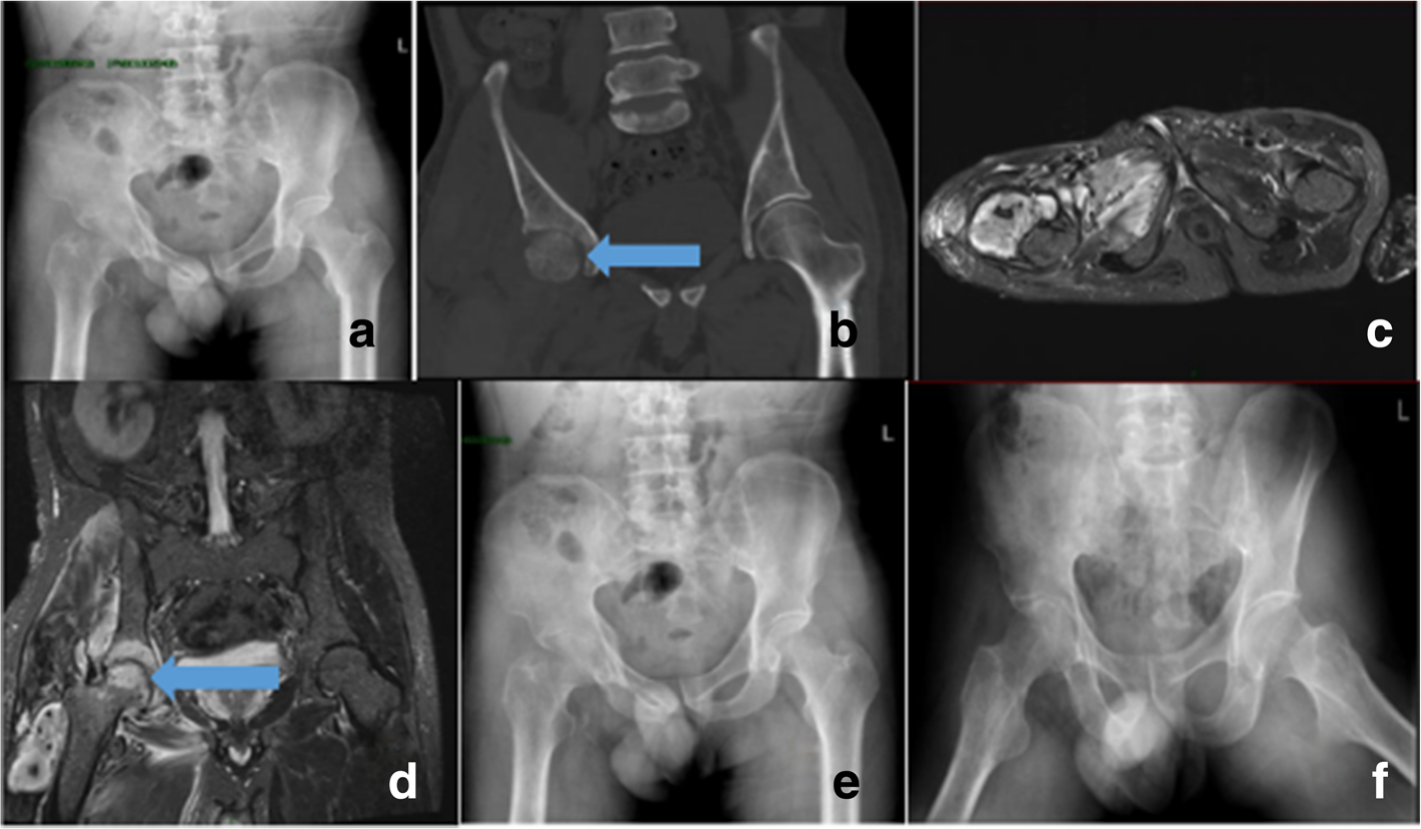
Patient 2: X-ray and computed tomography images of the pelvis showed bone destruction, subchondral erosion, and sclerosis (a, b). Magnetic resonance images of the right hip (c, d) showed marked joint effusion, synovitis, and soft-tissue swelling. Post-treatment X-ray images showed narrowing of the joint space and osteoporosis (e, f)
